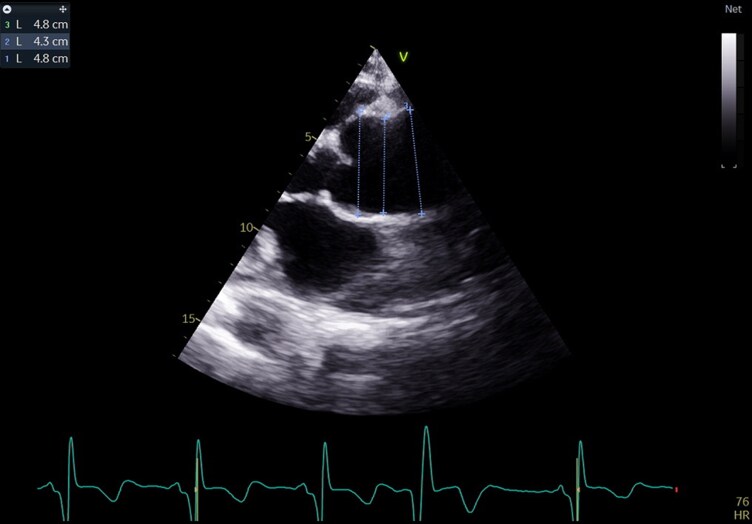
Parasternal long axis view in end-diastole. Dilation of the ascending and horizontal aorta: Valsalva sinuses = 48 mm, tubular junction = 43 mm, ascending aorta = 48 mm.
